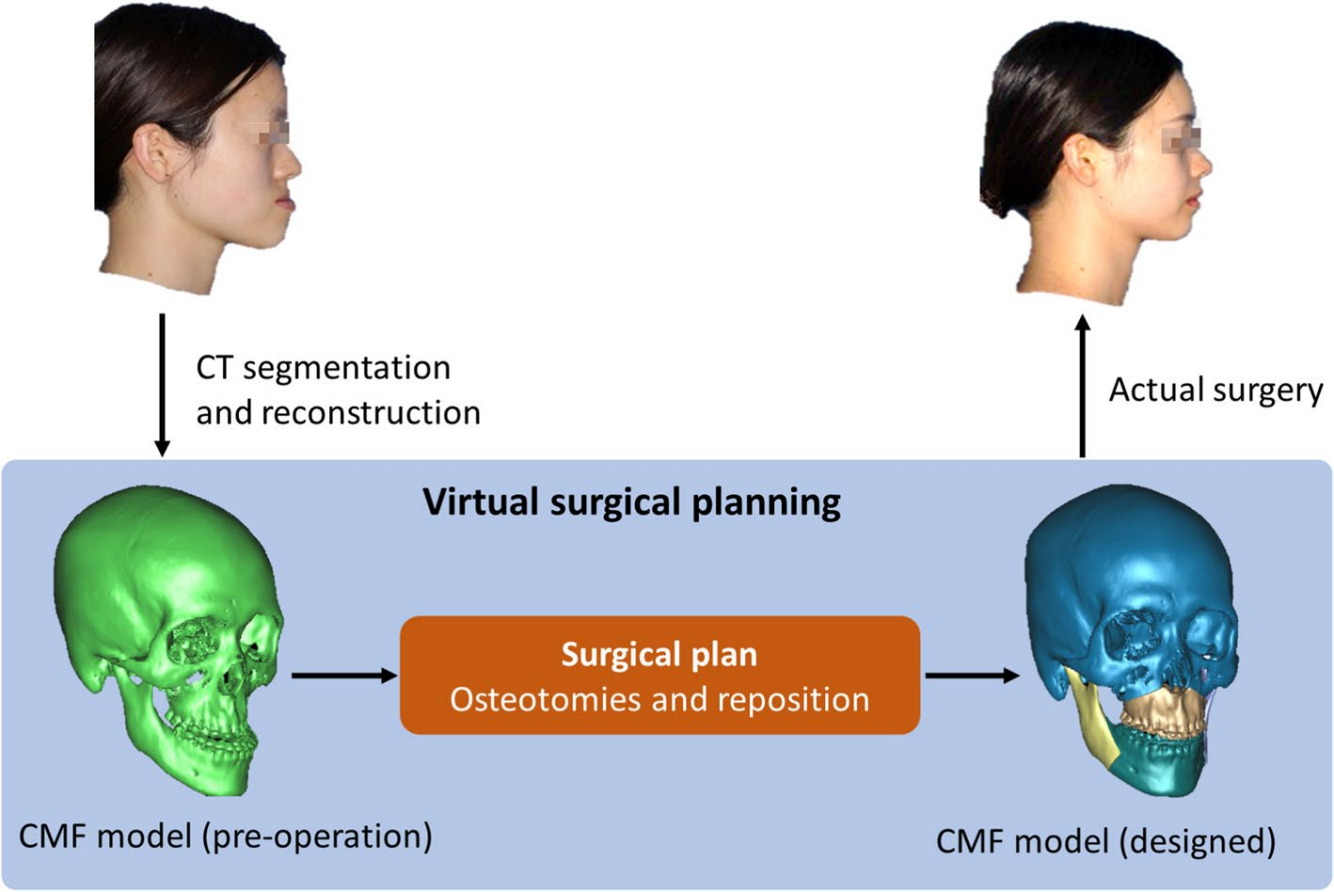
VSP procedures for orthognathic surgery. Before surgery, patient's computed tomography (CT) data were collected to reconstruct individual craniomaxillofacial (CMF) model. A patient-specific normal CMF structure can be designed through performing osteotomies on jawbones and repositioning bony segments. The determined jaws' position and occlusion can be transferred to actual surgery through surgical guides
